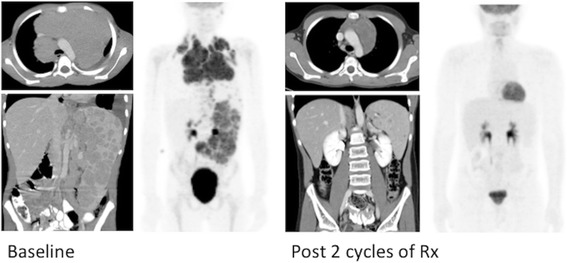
PET/CT in Hodgkin Lymphoma. Baseline PET/CT shows bulky mediastinal, extensive splenic and intra-abdominal nodal disease. Following 2 cycles of chemotherapy the ^18^F-FDG PET/CT shows a complete metabolic response to therapy, whereas residual mediastinal soft tissue mass and splenic lesions remain. Following a response-based treatment algorithm, subsequent therapeutic decisions are made based on the metabolic complete response (CR) shown by PET/CT
